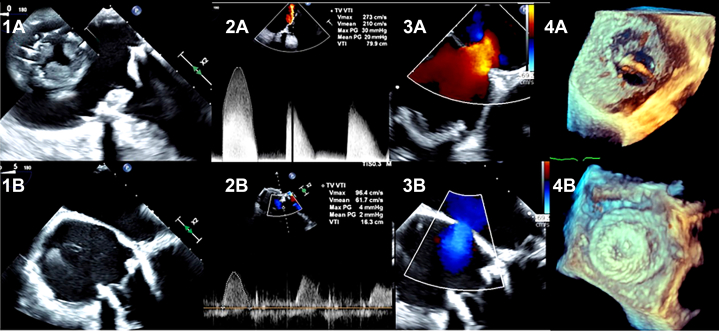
Transesophageal Echocardiography and Doppler Findings (1A) Prosthetic tricuspid valve thrombosis with severe stenosis and (1B) improved leaflet motion after valve-in-valve replacement. (2A) Preintervention continuous-wave Doppler across the tricuspid valve demonstrating severe obstruction (mean gradient: 20 mm Hg) and (2B) normalization postintervention (mean gradient: 2 mm Hg). (3A) Color Doppler showing right-to-left atrial shunt before tricuspid valve replacement and (3B) shunt reversal after valve replacement. (4A) Three-dimensional reconstruction of the interatrial septum before closure and (4B) after deployment of an Amplatzer septal occluder.
